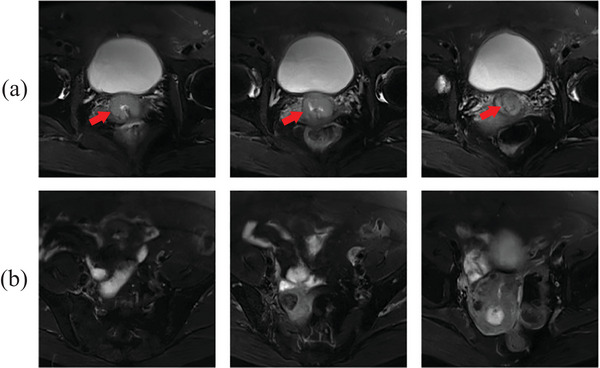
A failure example by our RA‐MIL. (a) MRI slices containing the tumor but excluded by our RA‐MIL. (b) MRI slices included by our RA‐MIL, but without tumor tissue. Red arrows in (a) point to tumor regions.
